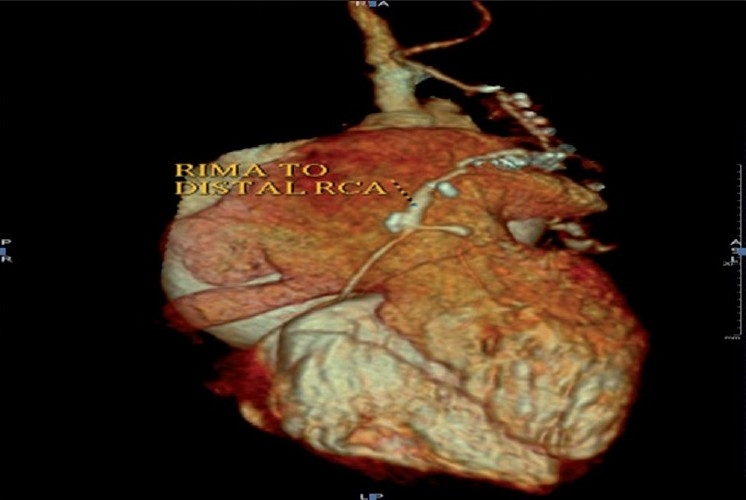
Postoperative CT angiogram picture showing the patent RIMA graft with interposed saphenous venous graft
